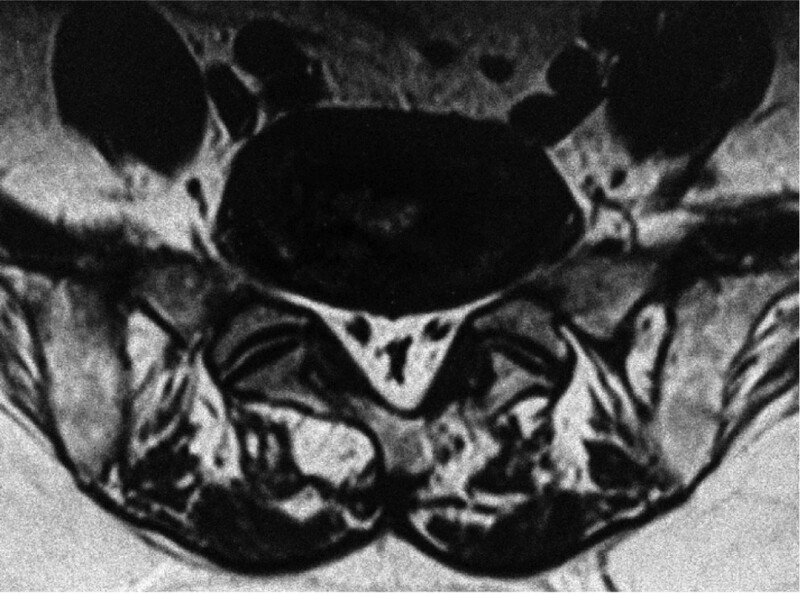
Our hospital treated an elderly male who suffered from neurogenic intermittent claudication. According to MRI imaging (axially) of the lumbar spine, fatty deposits are observed in the L5-S1 spinal canal, and there is a Y-shaped compression of the dural sac.^[19]^ MRI = magnetic resonance imaging.
